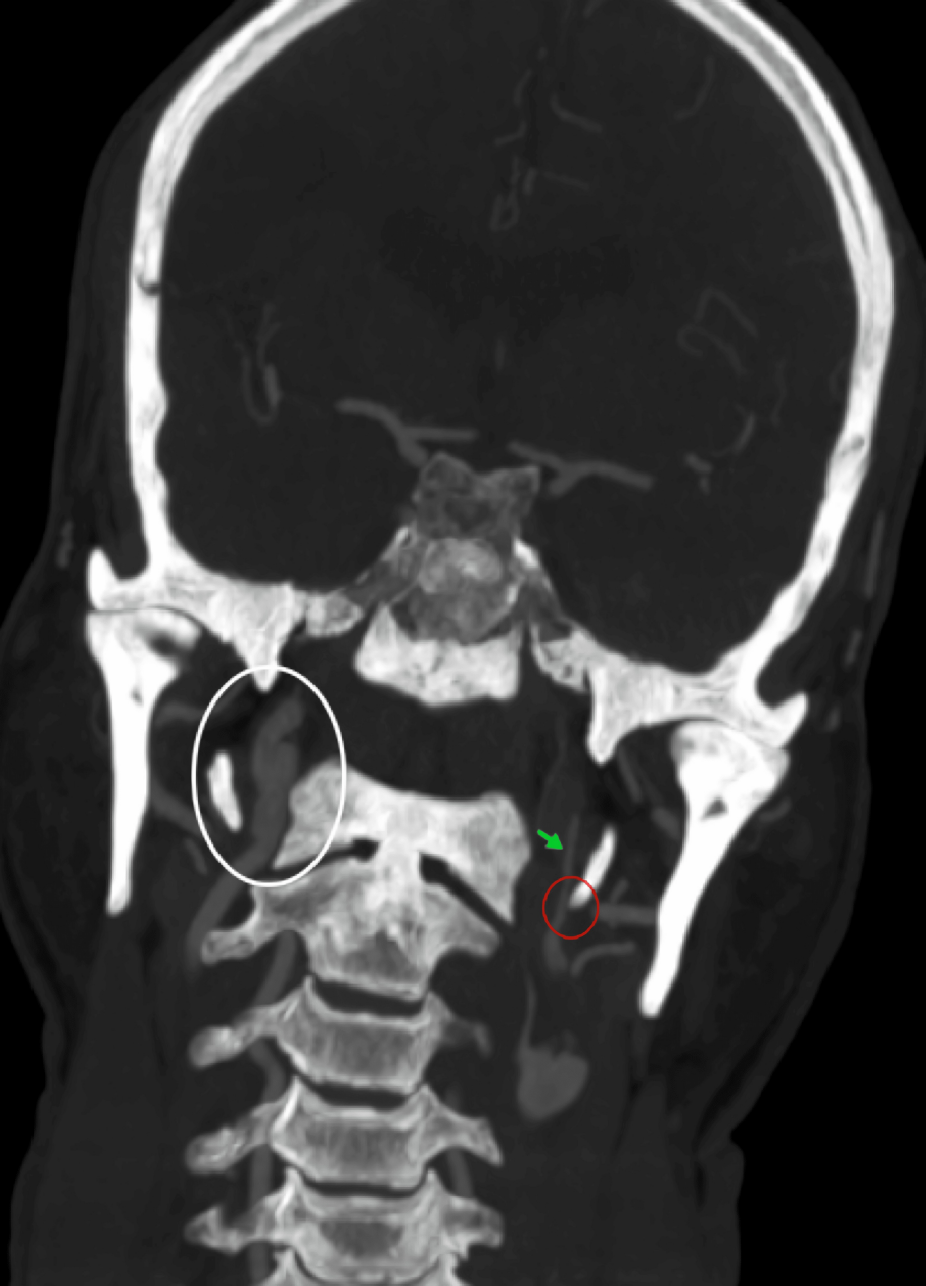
Computed tomography angiography (CTA) of the head and neck revealed a new left internal carotid artery (ICA) dissection (green arrow), resulting in severe stenosis of the ICA caused by the impinging elongated styloid process (ESP) (red circle). Persistent involvement of the contralateral ICA, including dissection and conflict with the right ESP, is shown within the white circle.
